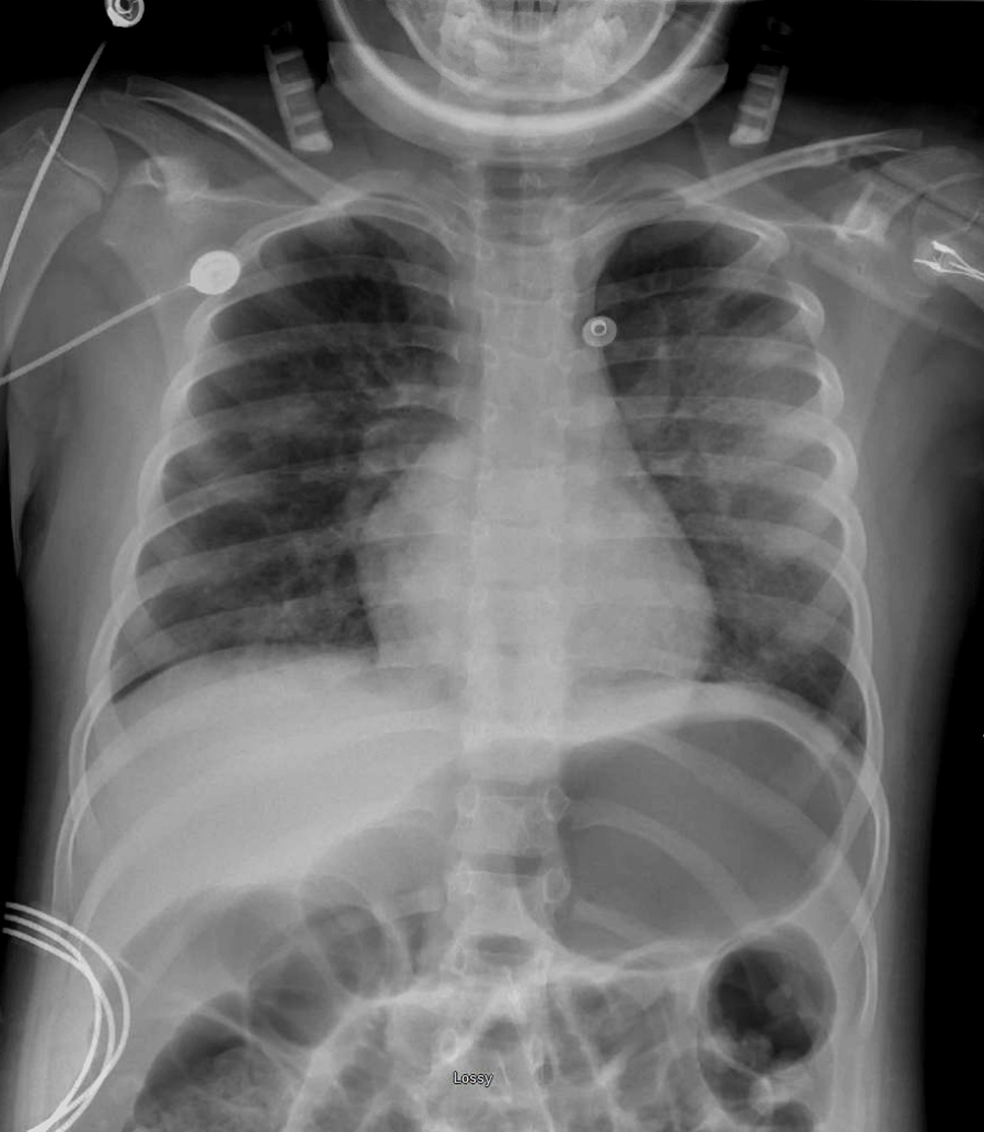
The patient's chest X-ray 10 hours later following BiPAP showed resolving pulmonary edema.
